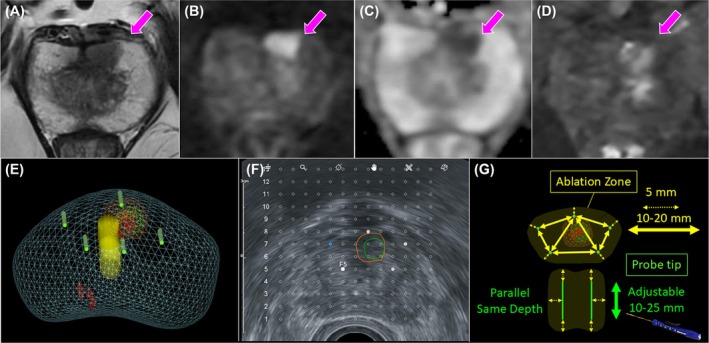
Representative case of image‐fusion‐guided irreversible electroporation. At baseline, the prostate multiparametric MRI ([A] T2‐weighted imaging, [B] diffusion‐weighted imaging, [C] apparent diffusion coefficient map and [D] dynamic contrast‐enhanced imaging) demonstrated a 43 cc gland with a PSA density of 0.1 ng/mL^2^ and a 13 mm PI‐RADS 4 lesion (pink arrow) in the left mid‐anterior prostate. (E) MRI/TRUS fusion‐guided transperineal prostate biopsy revealed GG 2 clinically significant prostate cancer localized to the left mid‐anterior lesion (red cores in the left lobe). A right‐sided systematic core demonstrated a small‐volume GG 1 insignificant cancer (red cores in the right lobe). (F) The IRE electrode placement through a grid template. The orange sphere indicates the MRI‐defined target boundary, whereas the green sphere represents the TRUS‐defined boundary. (G) Key considerations regarding treatment extent and spatial relationships during IRE electrode placement. GG, Gleason grade group; IRE, irreversible electroporation; MRI, magnetic resonance imaging; PI‐RADS, Prostate Imaging Reporting and Data System; PSA, prostate‐specific antigen; TRUS, transrectal ultrasound.
